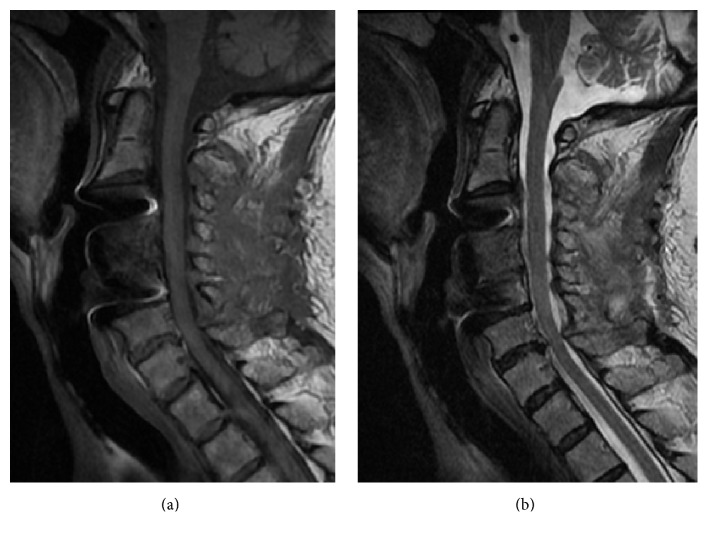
MRI taken three weeks after the second round of surgery. The spinal cord enlargement and intramedullary high intensity change disappear on T1 (a) and T2 (b) weighed MRI.
